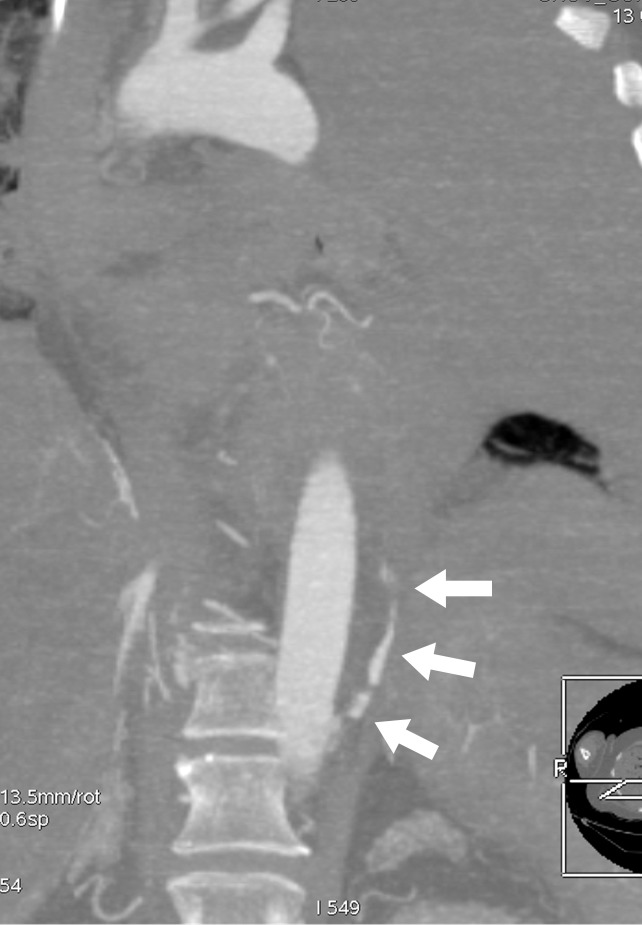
(a) Postmortem CT and (b–d) and arterial phase postmortem CT angiography images in a 59-year-old woman who died of internal exsanguination shortly after Whipple surgery. (a) A large left-sided hemothorax with mediastinal shift to the right. During the surgery, supraceliac clamping of the abdominal aorta was performed to stop intraperitoneal bleeding. The clamp was later loosened but left in place (arrow in b). The fatal hemothorax was caused by hemorrhage from the left 11th intercostal artery, which was torn near its origin from the aorta just above the diaphragm during placement of the clamp. The contrast media extravasation from the artery’s origin, reaching cranially into the thorax (arrows in b, c, and d), is displayed on arterial phase postmortem CT angiographic images (c and d). This finding may have been difficult to detect at autopsy because of the small size and location of the vessel. In this case it could not be displayed at autopsy because of large amounts of intraperitoneal and intrathoracic clotted blood, and multiple previous abdominal operations with extensive scar tissue formation and adhesions.
